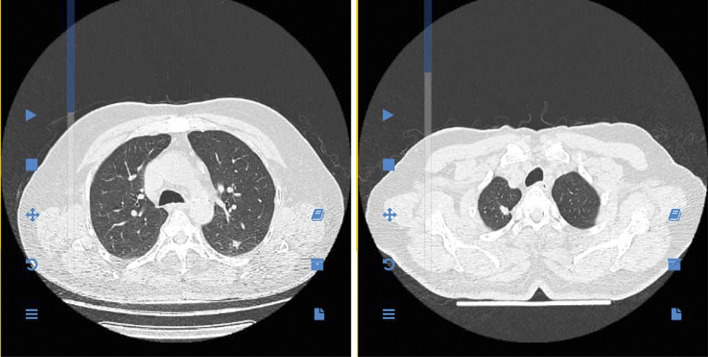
Axial chest CT showing bilateral pulmonary nodules.
